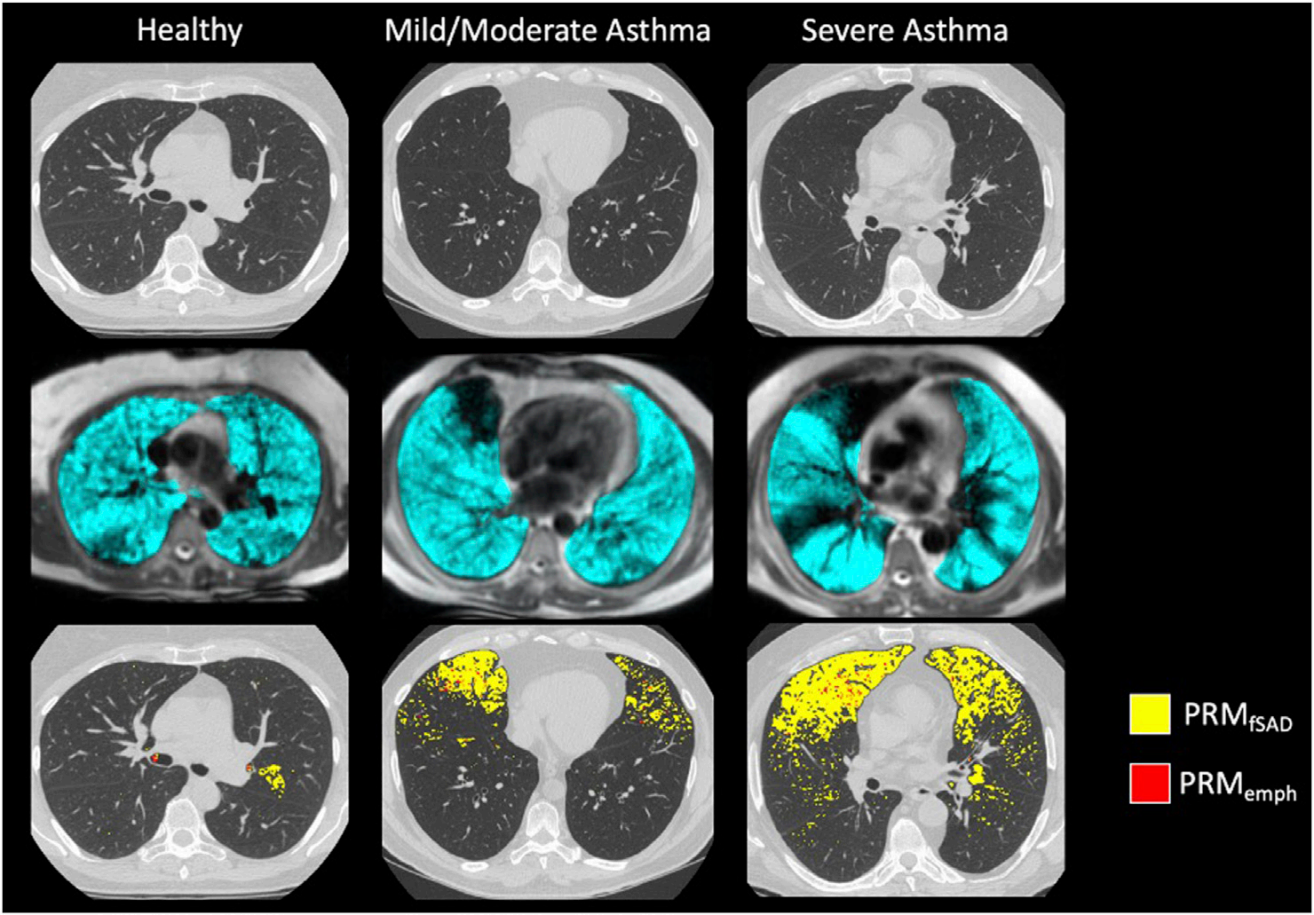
Example images of (from top to bottom): Inspiratory CT, HP 3He MRI overlaid on conventional MRI, and PRM maps overlaid on Inspiratory CT for a (from left to right) healthy subject, moderate asthmatic, and severe asthmatic.
